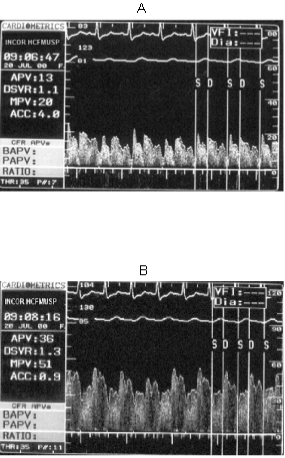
Right coronary artery spectral Doppler coronary flow velocity signal in baseline (A) and hyperemic (B) conditions. S = systolic, D = diastolic, portions of phasic coronary flow. APV = time-averaged peak coronary flow velocity. DSVR = diastolic/systolic flow velocity ratio.
